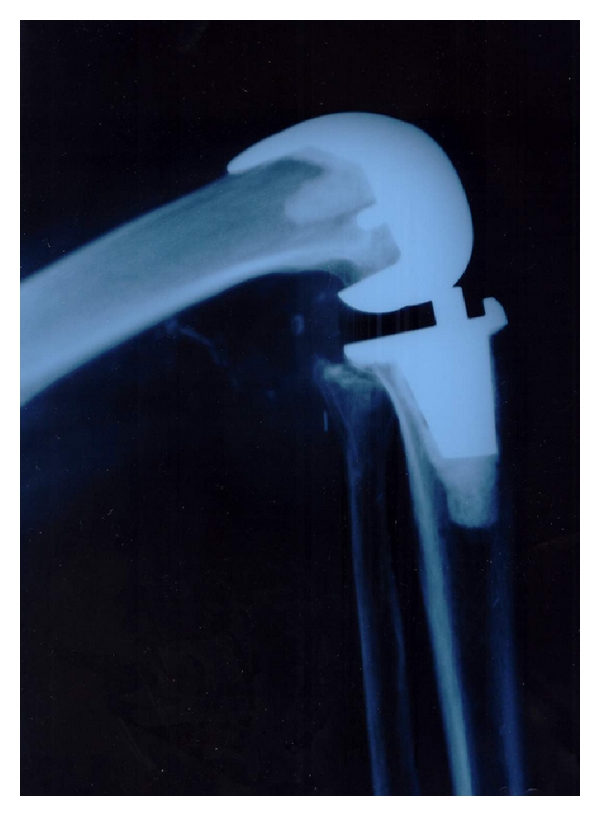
Lateral X-ray of a NexGen Full Flex implant in maximum flexion of the knee joint: patient does not reach the flexion limits of the implant. The dorsal implant condyles are some millimeters longer than the original bone condyles. (Notice the vessel clips in the calf, from earlier surgery).
